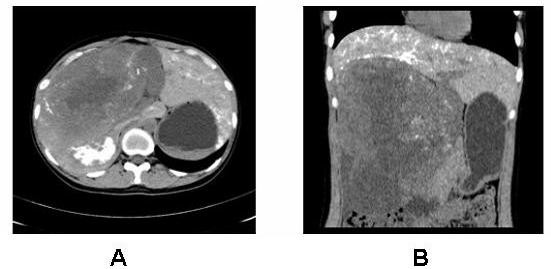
CT scan after the treatment of TACE shows that there is almost no lipiodol deposits in the tumor area.
